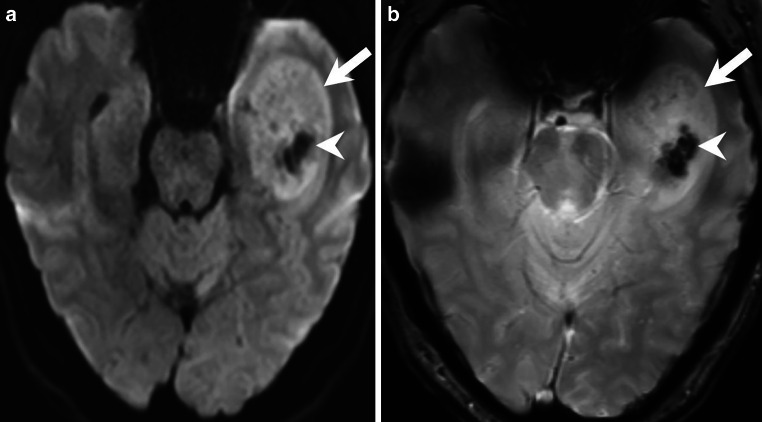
On axial diffusion-weighted images (b-value: 1000, a), the lesion showed signs of slightly restricted diffusion (*arrow*). Note the central and well-demarcated signal loss (a, *arrowhead*). On axial T2*-weighted images (b), this area within the lesion (b, *arrow*) also appeared homogeneously hypointense (b, *arrowhead*). This area could correspond to either local calcification or intralesional hemorrhage. Based on the MR images, we could not reliably distinguish the two. Unfortunately, we did not perform a preoperative computed tomography (CT) scan
